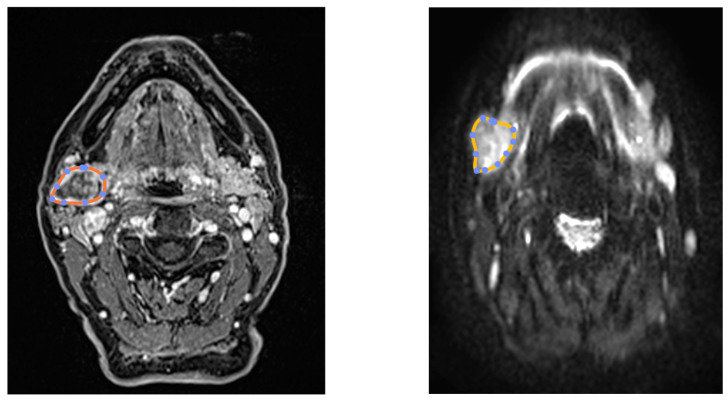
Segmentation of dynamic DCE and DWI.
